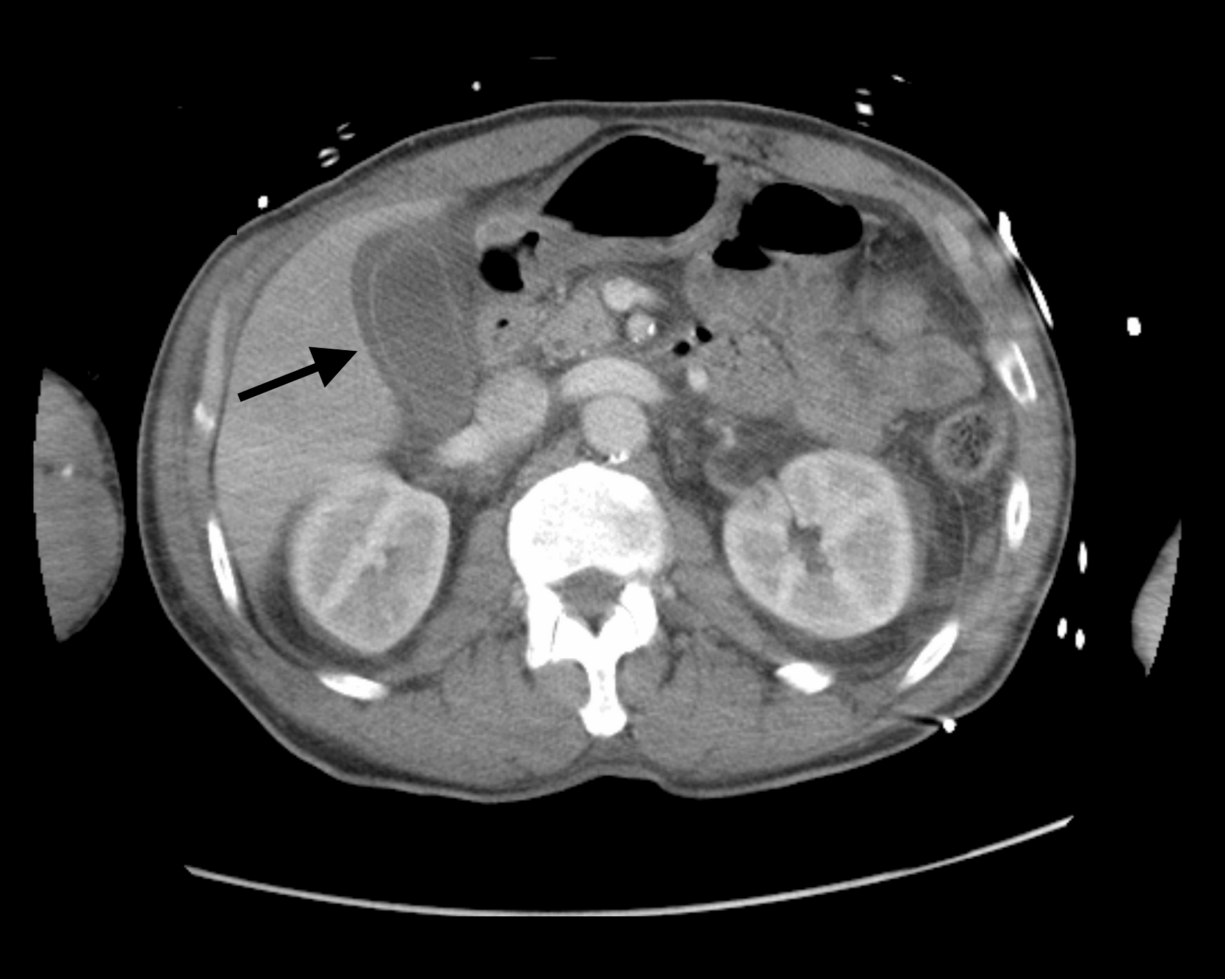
Axial CT scan of the abdomen and pelvis demonstrating abdominal edema, mild ascites, and trace bilateral pleural effusions without evidence of abscess, hematoma, or hydronephrosis (black arrow).
